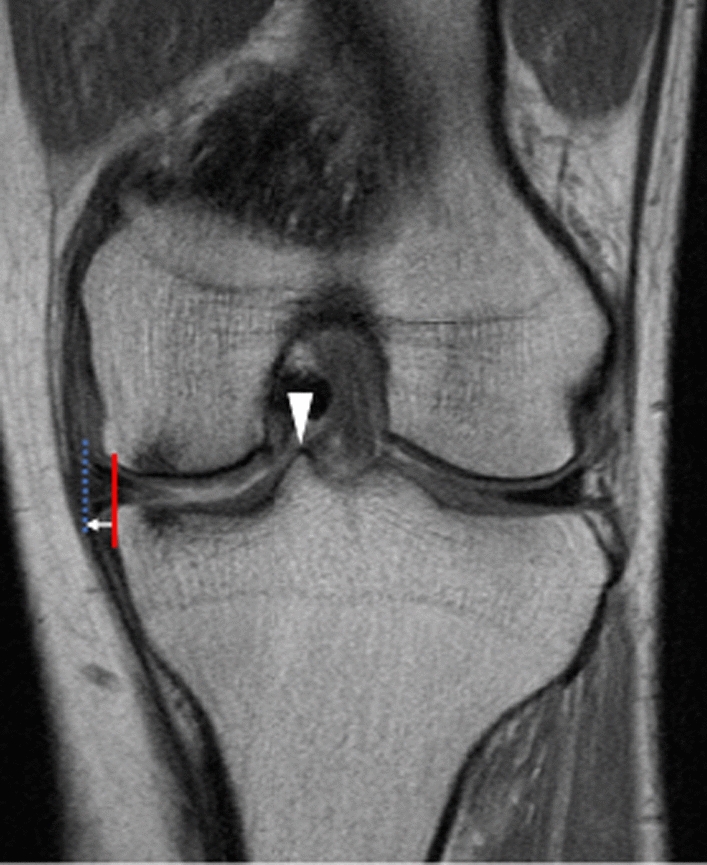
MME measurement. MME (white arrow) was measured as the distance between the medial edge of the MM (blue dotted line) and the tibial plateau excluding the osteophytes (red line) in the slice in which the medial tibial eminence was the highest (white arrowhead) using MRI coronal images. MM, medial meniscus; MME, medial meniscus extrusion; MRI, magnetic resonance imaging
